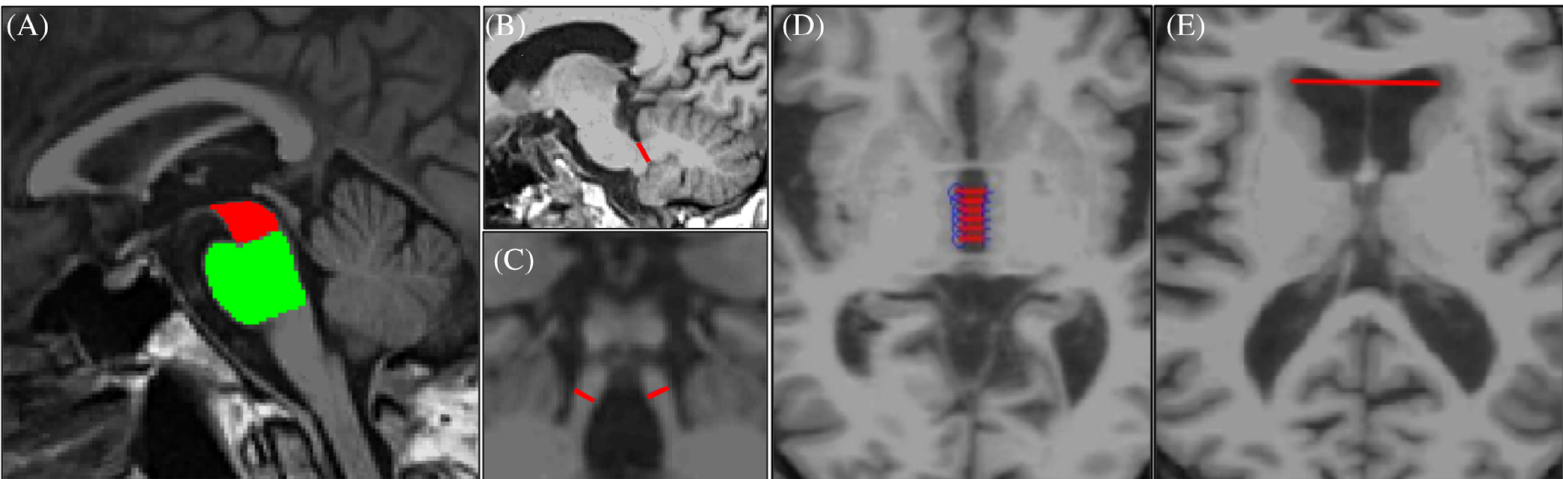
Automated measurement of the midbrain and pons area (A), the middle cerebellar peduncle width (B), the superior cerebellar peduncles width (C), the third ventricle width (D), and the maximum frontal horns width (E) on T1‐weighted magnetic resonance images. [Color figure can be viewed at wileyonlinelibrary.com]
